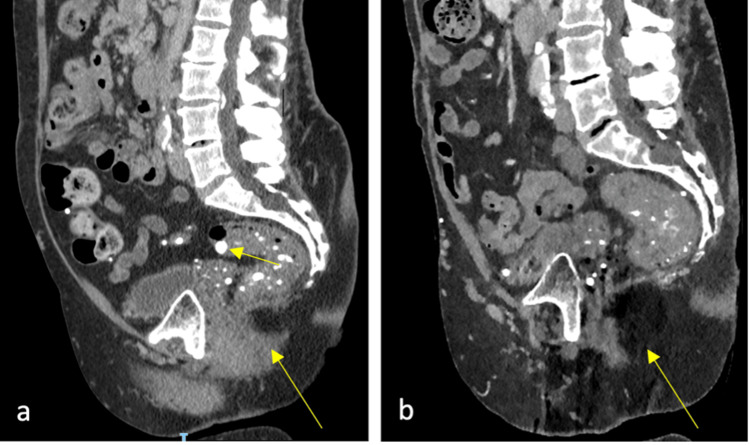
Sagittal sections of pelvic CT before (a) and after (b) Hartmann's operation, showing several vascular lesions within the pelvis (small arrow) together with the rectal stump in situ and resolution of the rectal prolapse postoperatively (large arrow).
